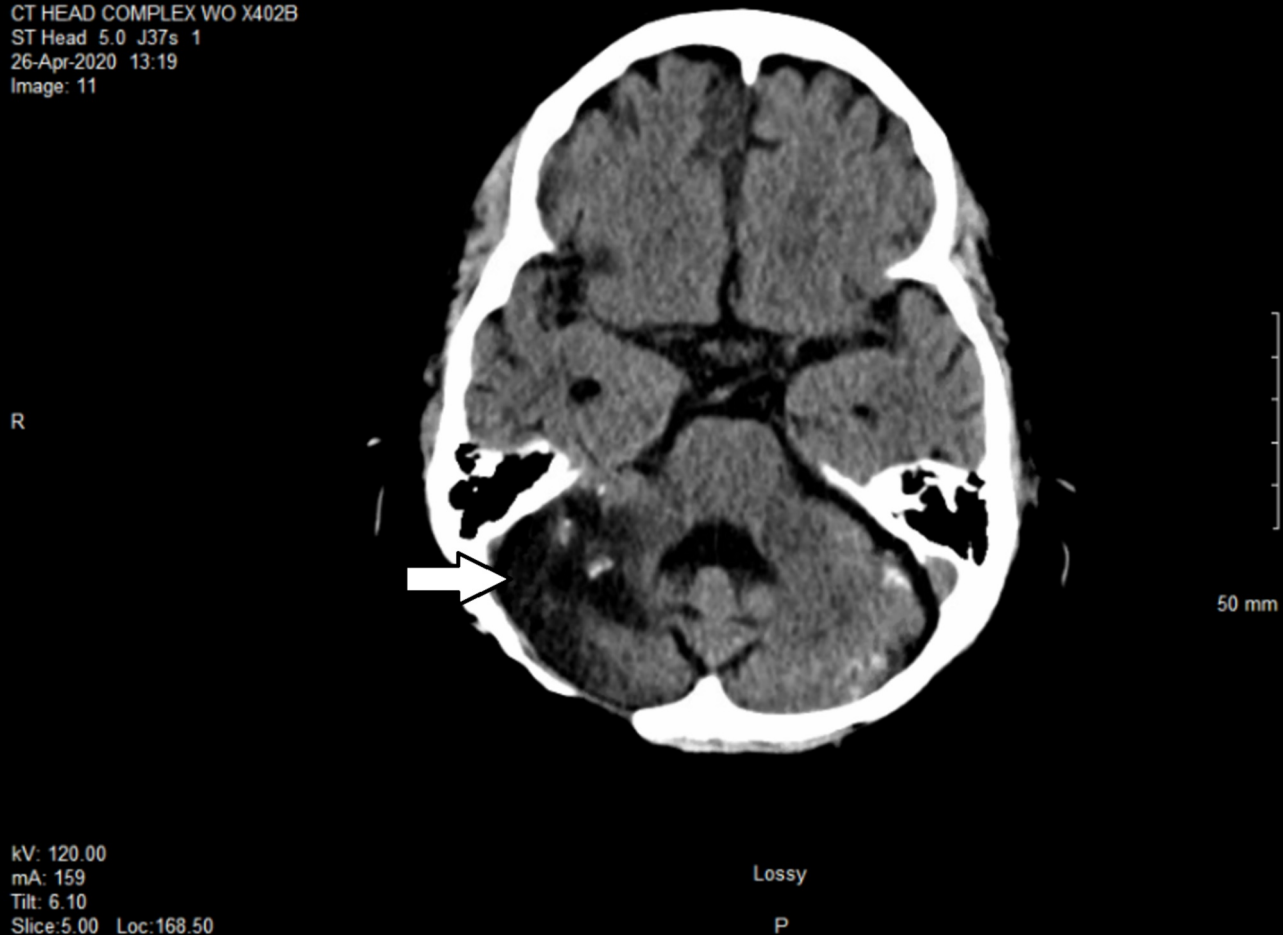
Brain CT showing near-complete response nine months after HSRT HSRT, hypofractionated stereotactic radiotherapy
